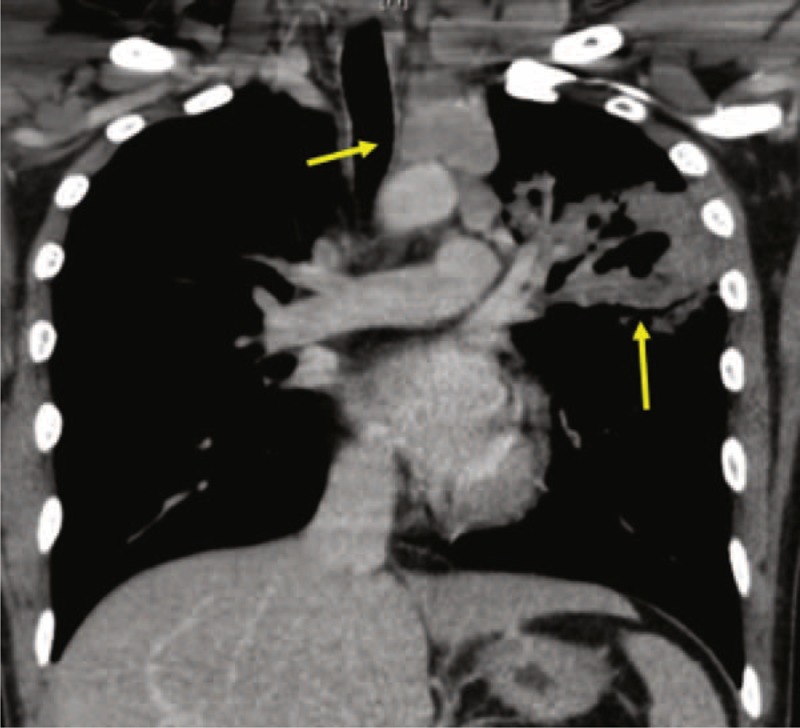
A representative case of patients with concomitant peripheral and central lesions undergoing both bronchial washing with biopsy, and EBUS-TBNA. Higher diagnostic yield was seen with EBUS-TBNA. EBUS-TBNA = endobronchial ultrasound-transbronchial needle aspiration.
